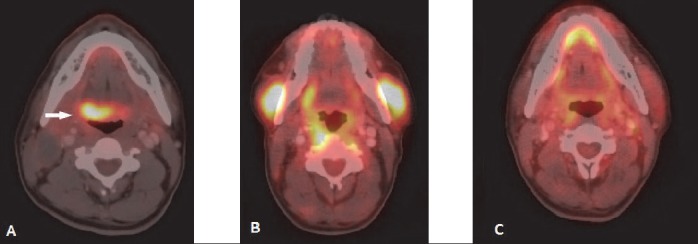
Lymphoma of tongue: (A) FDG avid plaque like lesion is seen in posterior tongue on right side (arrow). (B) Post 3 cycle chemotherapy shows partial metabolic response. Diffuse FDG uptake of both submandibular glands indicates chemotherapy induced sialadenitis. (C) Follow up scan few weeks after completion of 6 cycle of chemotherapy shows complete metabolic response. Both submandibular glands show normal appearance
